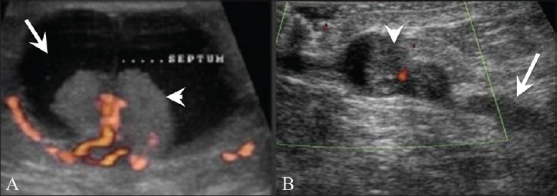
Intracystic (A) and intraductal (B) papillomas. Breast USG with color Doppler (A) in a 40-year-old with a palpable lump and bloody left nipple discharge shows a complex cyst (arrow) with a vascular, intracystic, solid polypoidal mass (arrowhead). A diagnosis of intracystic papillocarcinoma was made on excision biopsy. Breast USG (B) in a 60-year-old patient with a history of left-sided bloody nipple discharge shows a focally dilated duct (arrow) with an intraductal solid echogenic mass (arrowhead)
